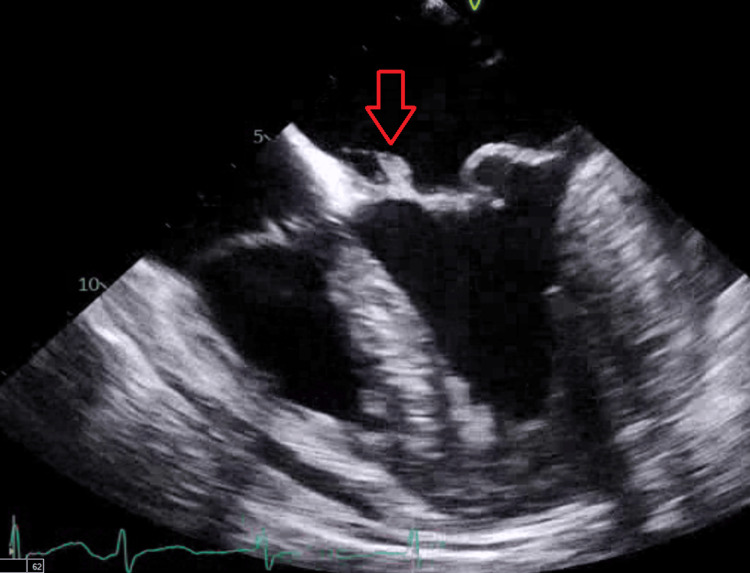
New vegetation on the mitral valve seen on transesophageal echocardiography. The red arrow indicates a new digitation on the mitral valve.
